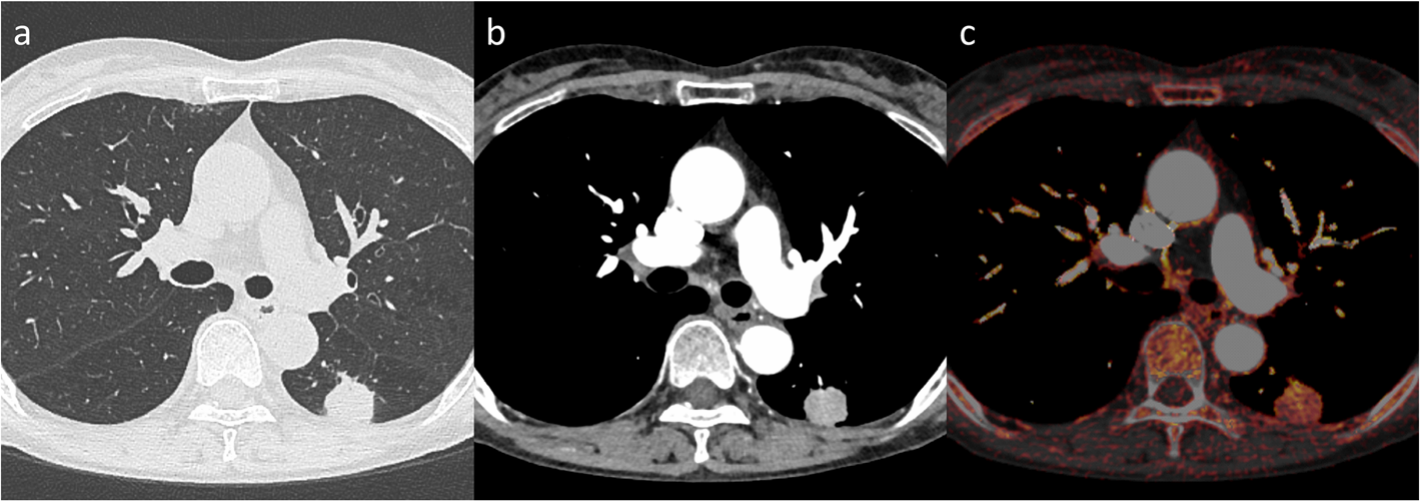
A 75-year-old female patient. A squamous cell carcinoma (pT1bN0) is observed in the left lower lobe. a Lung window setting. b Mediastinal window setting at the early phase of contrast-enhanced CT. c Iodine-enhanced image in contrast-enhanced CT. The 3D-IRA is 48 HU, and the corrected 3D-IRA is 6.45. The patient was recurrence-free for 51 months after a left lower lobectomy
